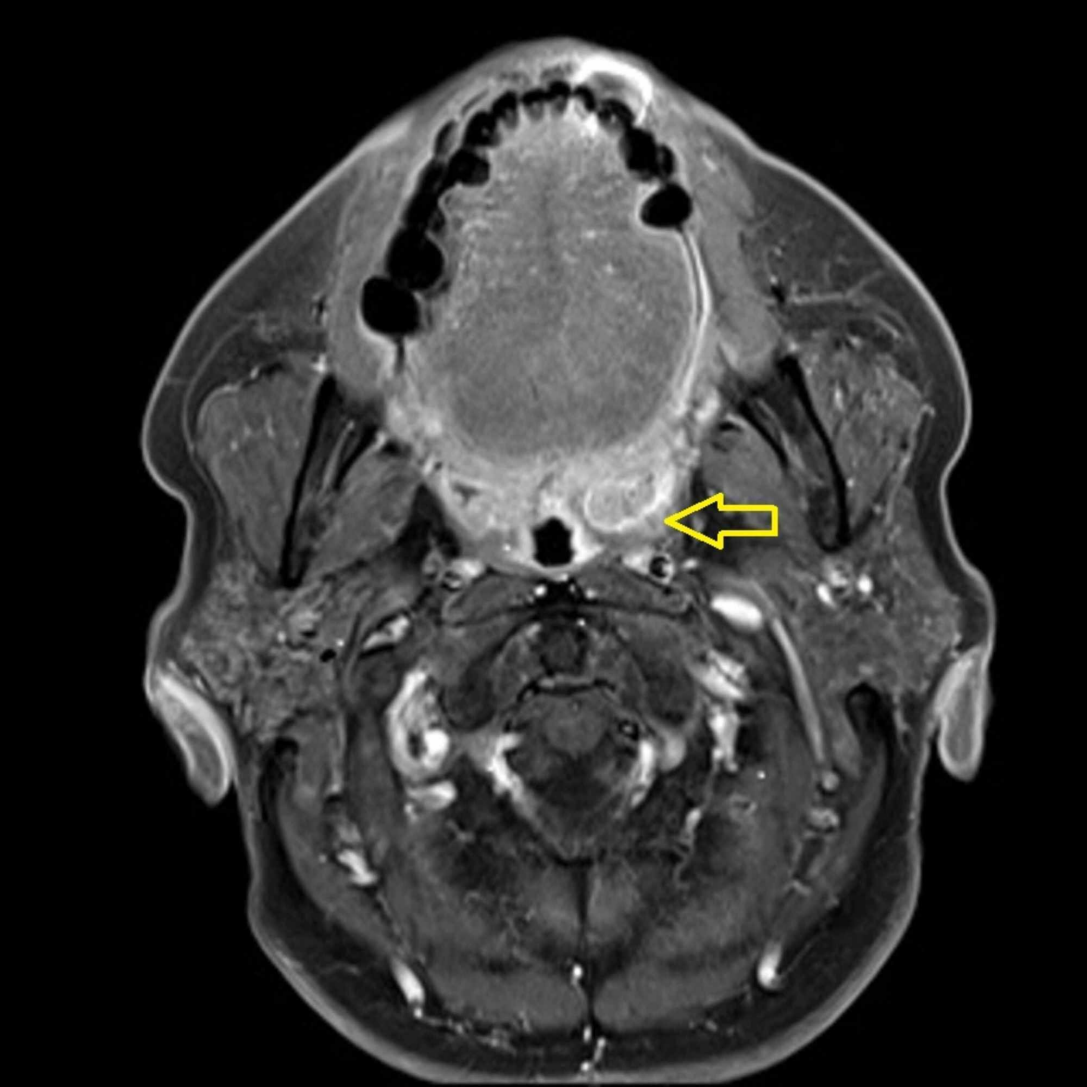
Case 2; head and neck MRI before CRT The tumor is located on the left tonsil; size is about 3 cm MRI: magnetic resonance imaging; CRT: chemoradiotherapy
